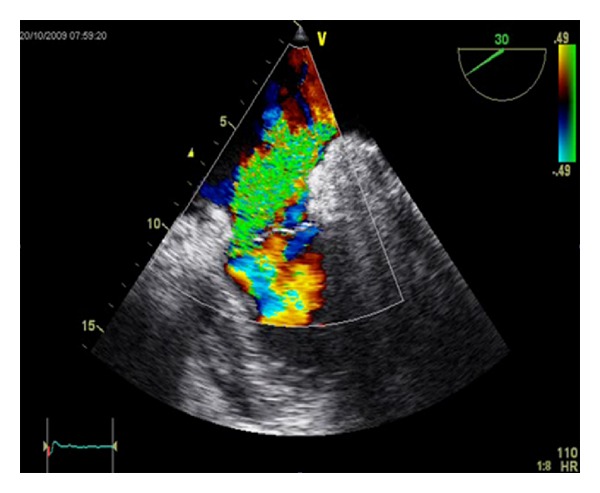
Echocardiogram shows severe mitral valve regurgitation.
